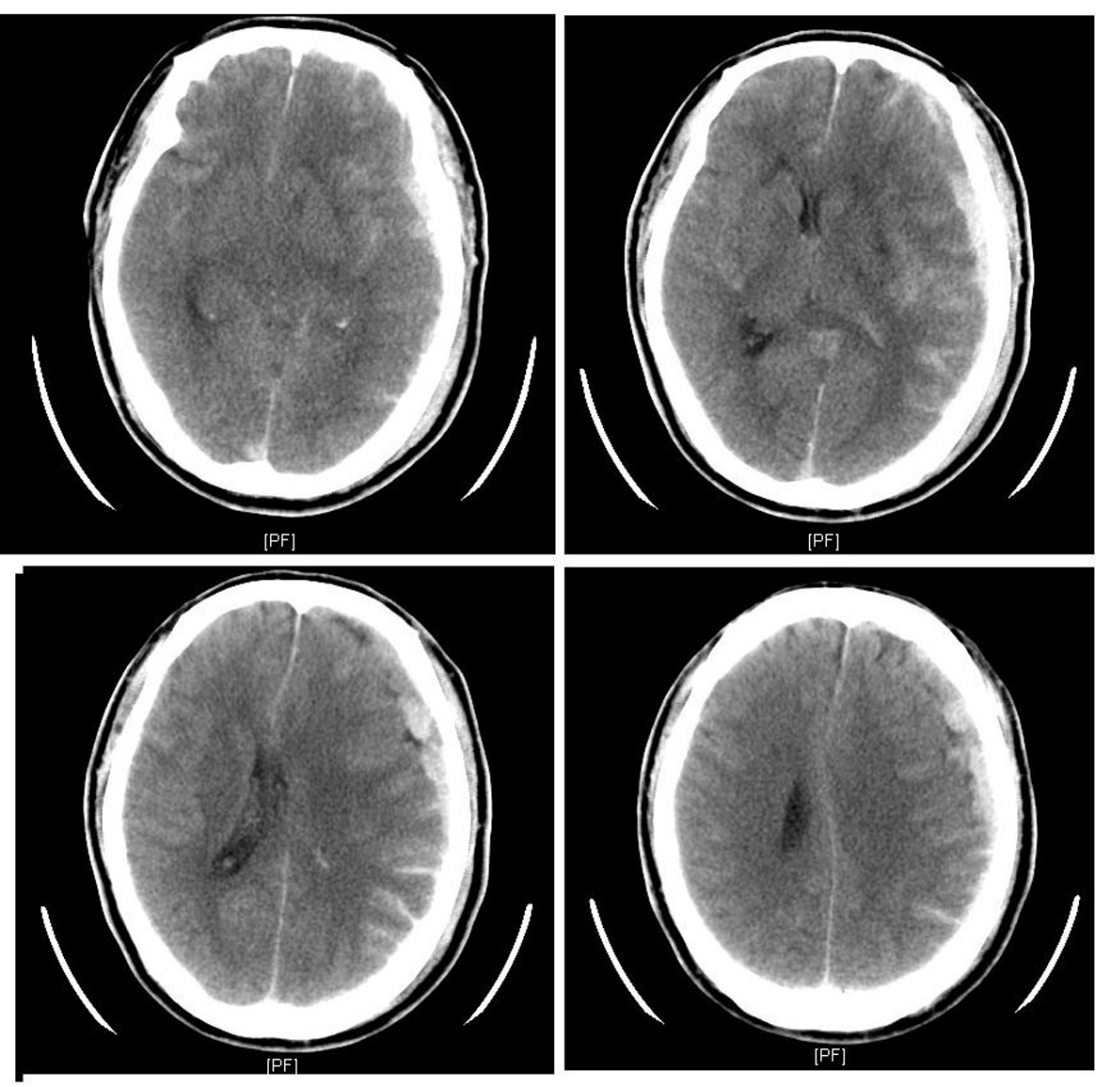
Head CT showed left subdural hematoma with subarachnoid hemorrhage and midline shifting to right side.
